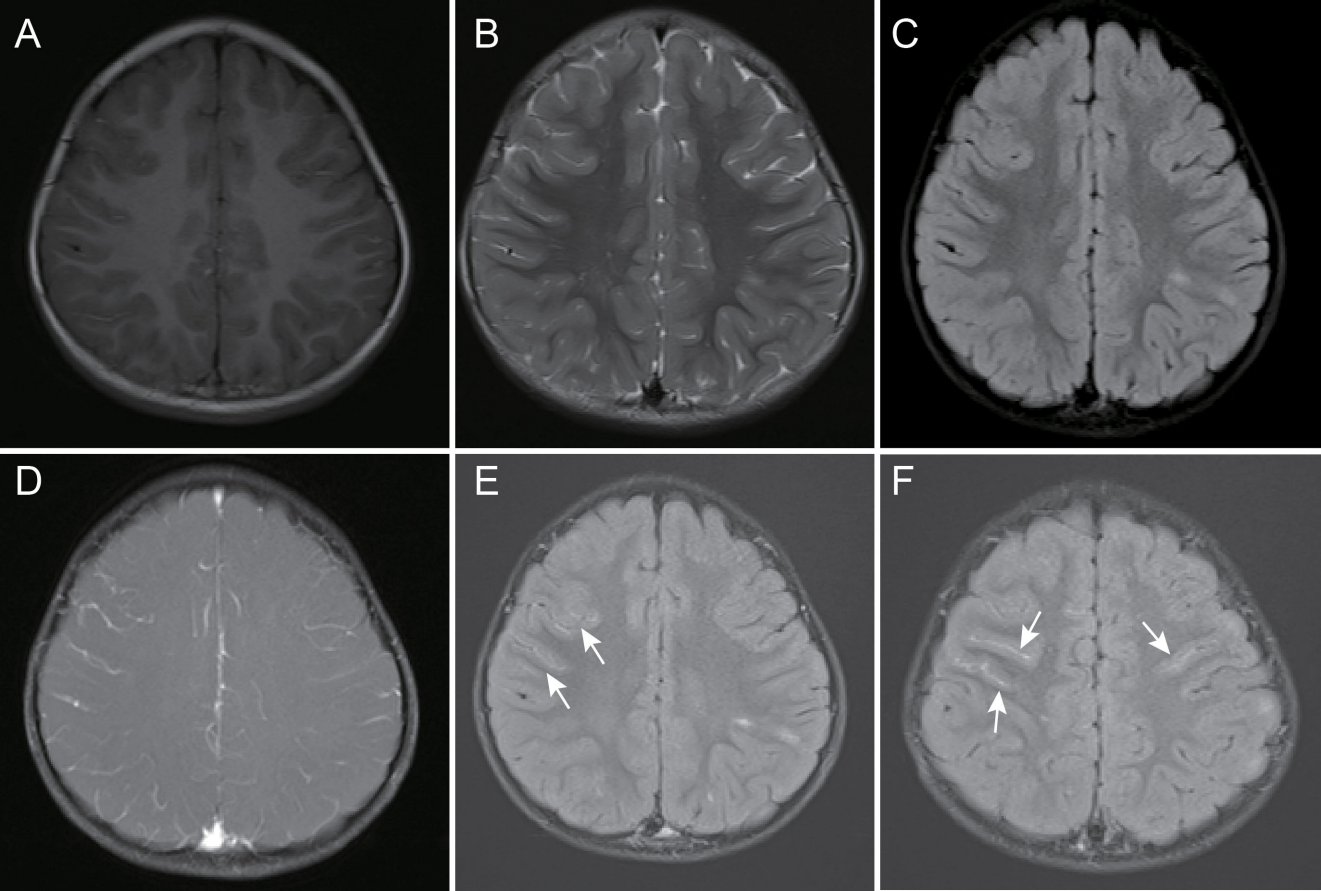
A 39-month-old female child with myelin oligodendrocyte glycoprotein antibody-associated encephalitis had a 2-day history of fever, headache, and emesis, accompanied by an episode of convulsion. Magnetic resonance imaging showed localized abnormal signal in the left parietal lobe juxtacortical white matter, and cortical gray matter, slight hypointensity on T1-weighted images (A), hyperintensity on T2-weighted and fluid-attenuated inversion recovery images (B, C), and thickening of blood vessels in the cerebral sulci of the right frontal lobe on contrast-enhanced fat-suppressed T1-weighted images and unobvious leptomeningeal enhancement (D) but partial bilateral leptomeningeal enhancement on contrast-enhanced fluid-attenuated inversion recovery images (E, F), arrow).
